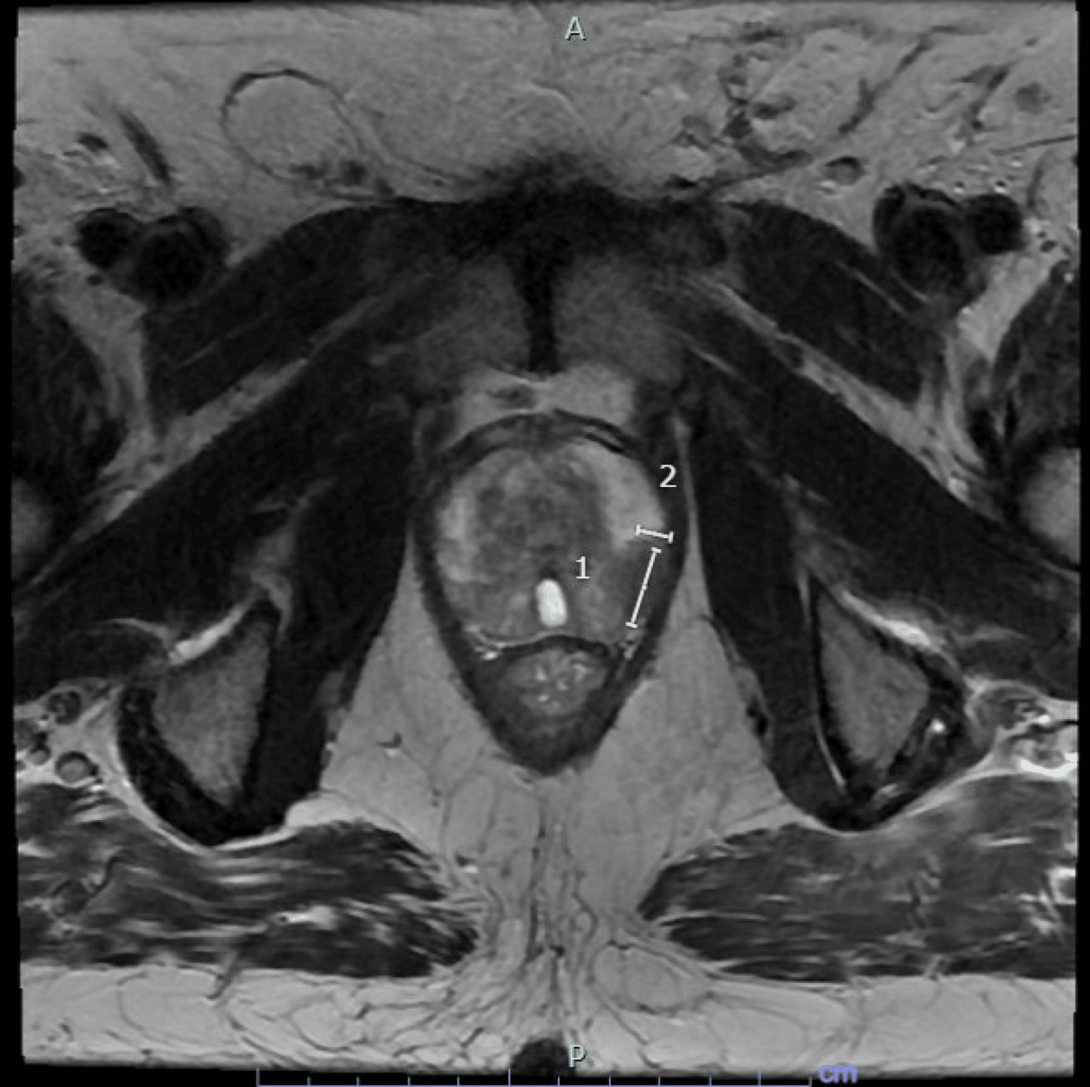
MRI of the prostate showed a lesion in the peripheral zone at the mid-gland (the length of the lesion is labeled as “1” and the width is labeled as “2”). MRI = magnetic resonance imaging
